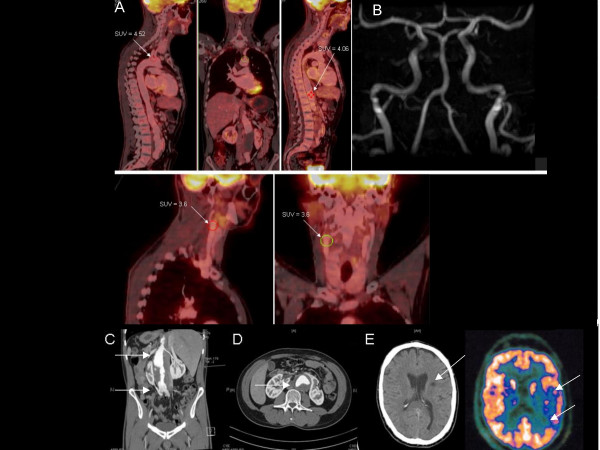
FDG PET-CT, MR-angiography and CT in a patient with TA and stroke. (A) F-18-fluorodeoxyglucose positron emission tomography-computed tomography showing fluorodeoxyglucose accumulation in the carotid arteries, ascending aorta, and the abdominal aorta cranial to the aneurysm (arrows with corresponding maximal standardized uptake values). (B) Magnetic resonance angiography of the cerebral vessels. (C), (D) Infrarenal aortic aneurysm (13 cm × 4.8 cm) with mural thrombus (arrows). (E) Computed tomography and fluorodeoxyglucose positron emission tomography revealing middle cerebral artery stroke (arrows).
